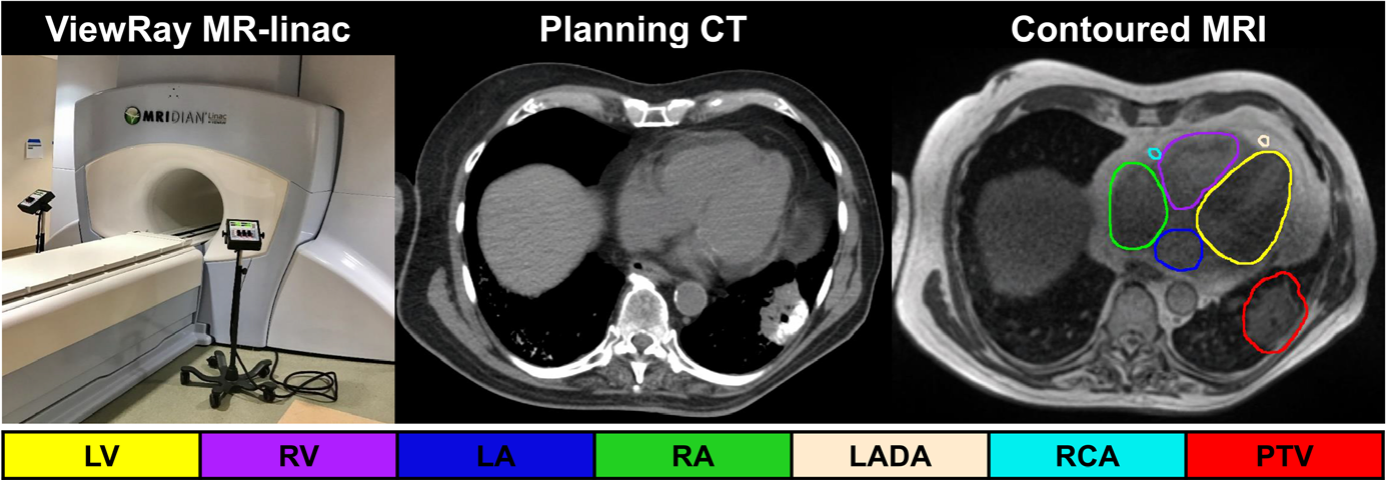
(Left) ViewRay 0.35T MR‐linac, (middle) treatment planning CT, and (right) 0.35T MR dataset with cardiac substructure contours evident and delineated. PTV: planning target volume (malignant neoplasm of lower left lung bronchus). Cardiac‐related abbreviations are defined in the text.
